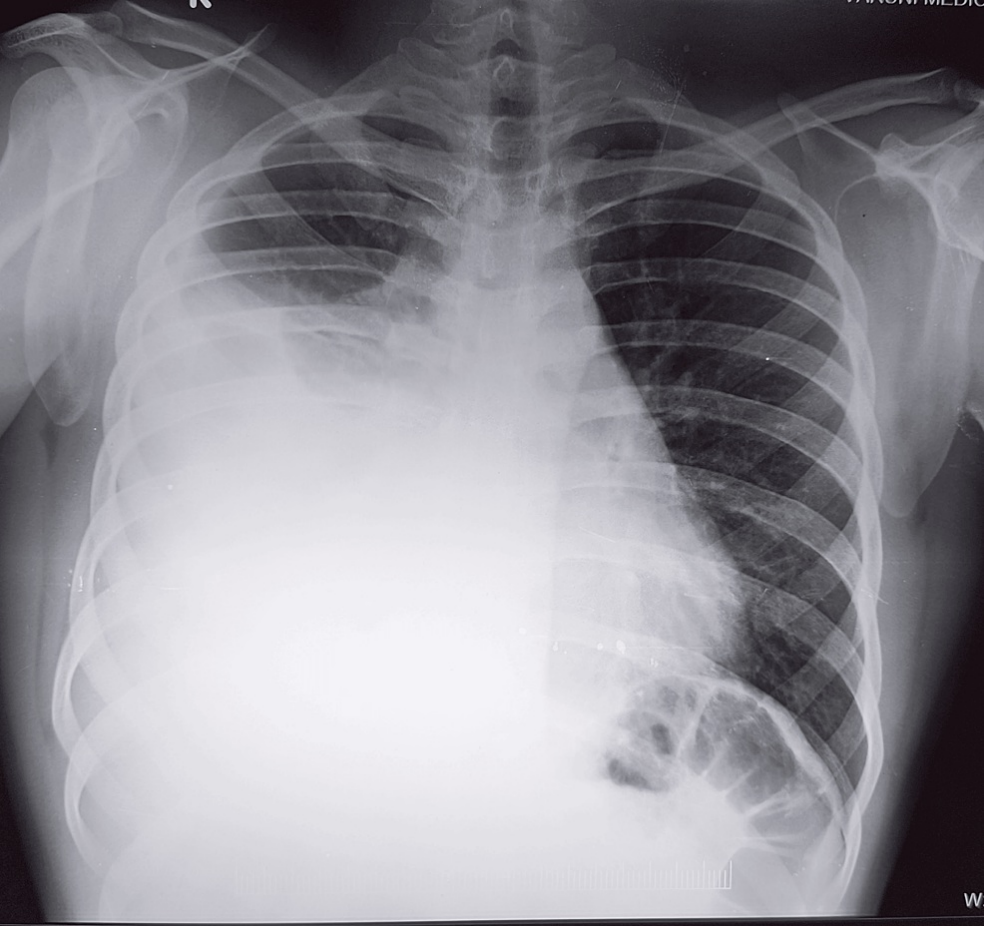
Initial chest X-rays revealed right costophrenic angle obliteration with a meniscus sign.
